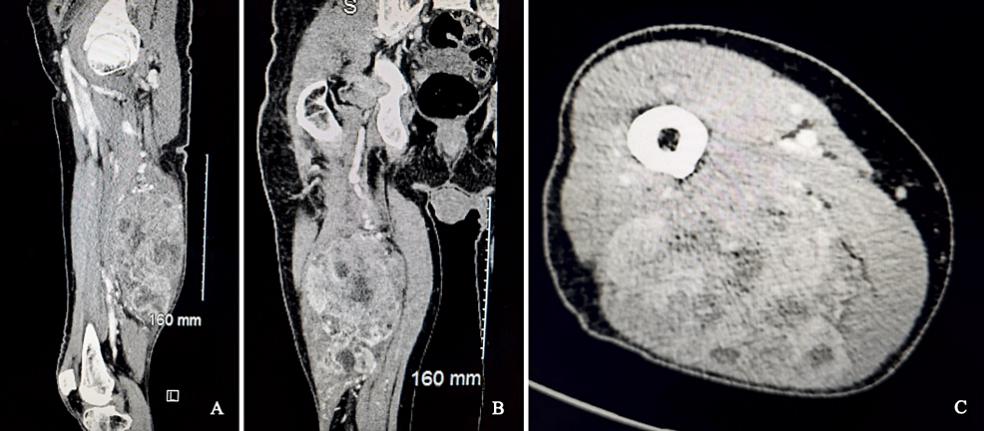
Computed tomography (CT) images. Sagittal (A), coronal (B), and axial (C) CT images of the right lower extremity with intravenous contrast demonstrate a 16.0 × 8.1 × 9.5 cm heterogeneously enhancing mass in the right hamstring muscle.
